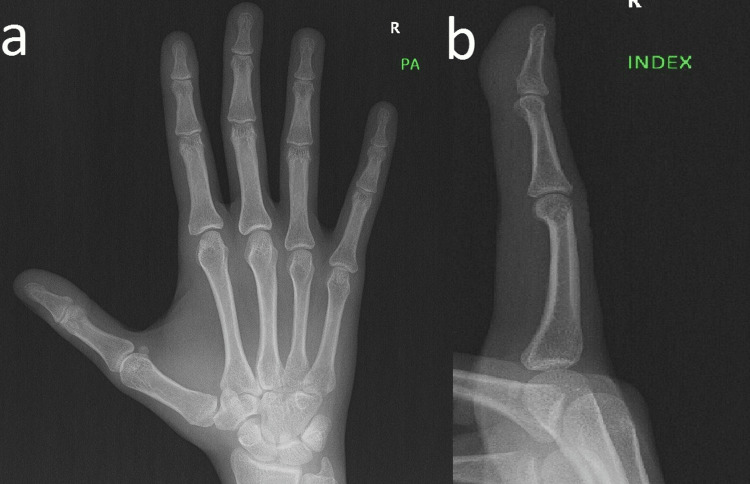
Posteroanterior X-ray of the right hand (a) and lateral view of the right index finger (b) Showing normal bones (no radiographic abnormalities) and soft tissue swelling in the bulb of the index
